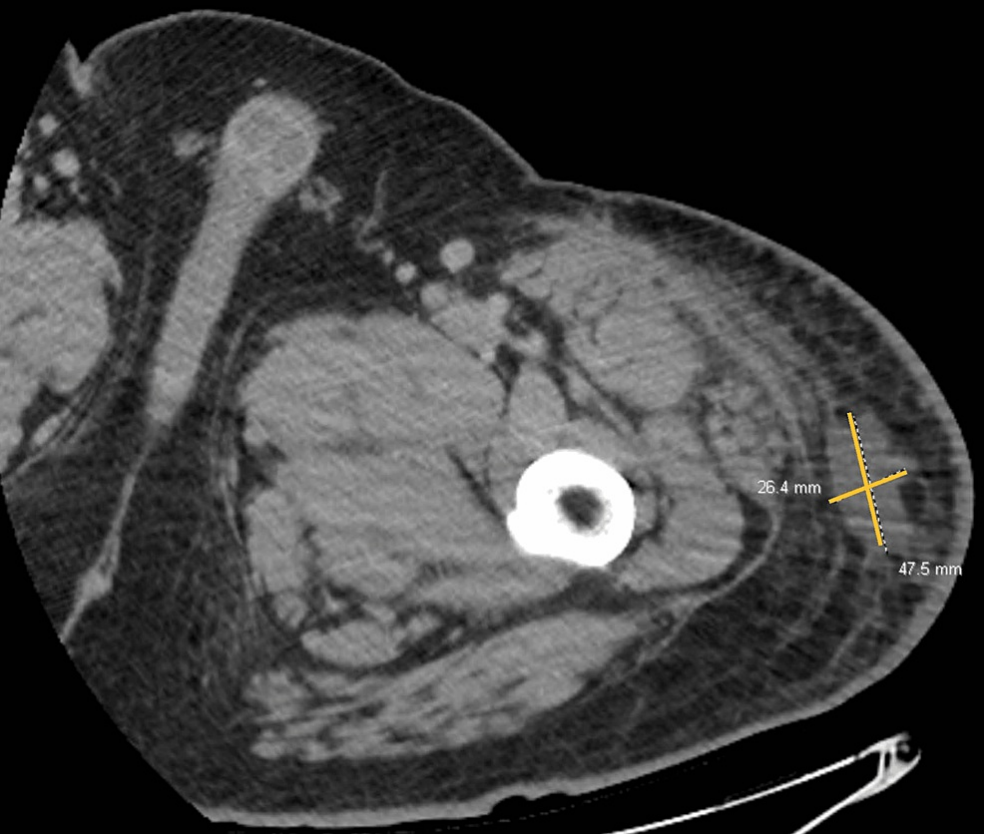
November 2022 CT left lower extremity with contrast status post-irrigation and debridement of left anteromedial thigh hematoma shows residual collection of gas and fluid measuring 4.8 x 2.1 x 40.0 cm, previously 13.6 x 5.4 x 43.4 cm. This image shows residual fluid collection 4.8 x 2.1 cm in the transverse dimension.
